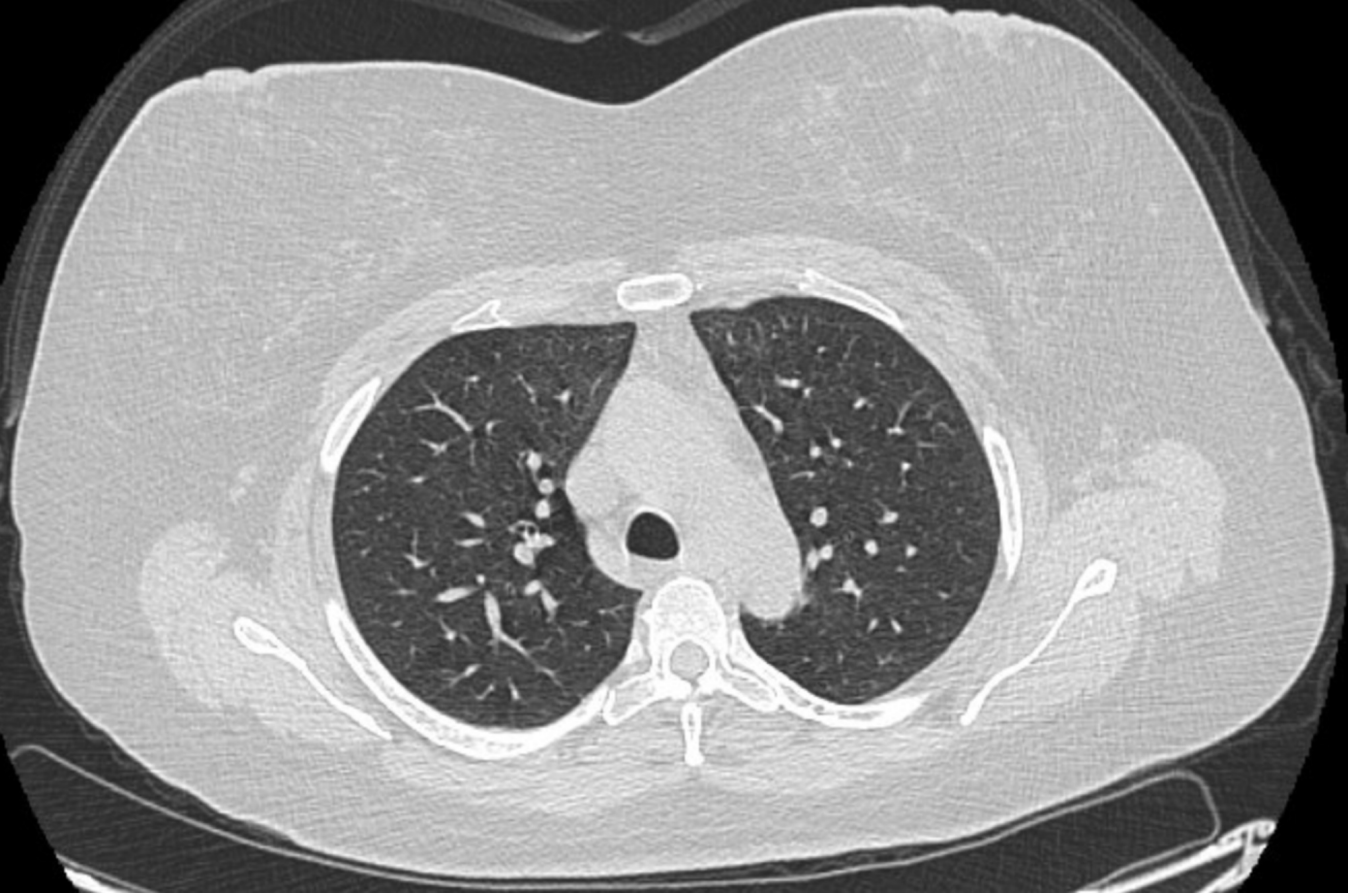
Follow-up CT chest after two months CT: Computed tomography
